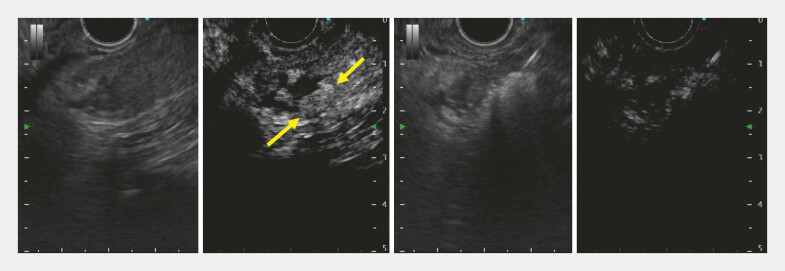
(Left) Contrast-enhanced endoscopic ultrasound visualized the residual tumor (arrow). (Right) Additional endoscopic ultrasound-guided ethanol ablation was performed on the residual tumor.
